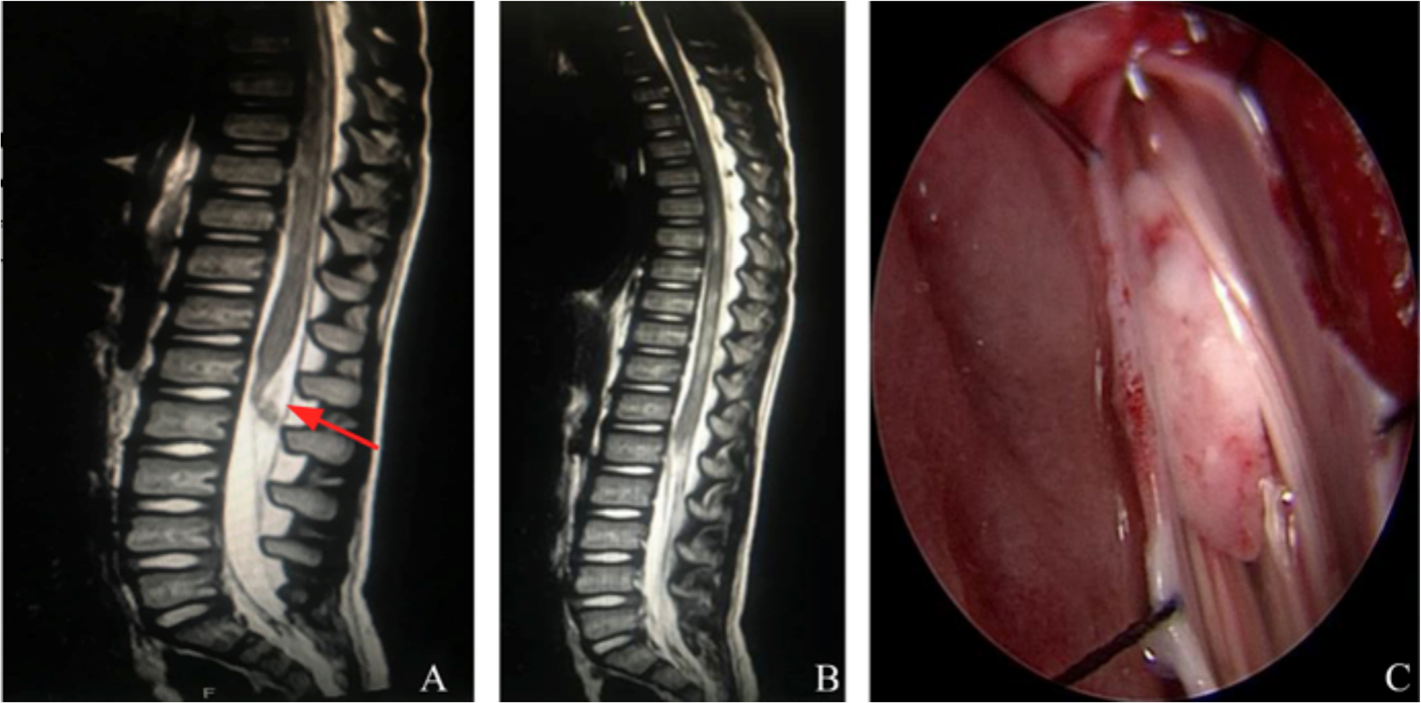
a A nodular T2 isointensity shadow was showed at the dorsal of the conus, which was located at L1 level with moderate swelling. b The sagittal MRI images showed longitudinally extended intramedullary patchy T2 hyperintensity at the T9-L1 level. c There were contusion and laceration in the cone and some contusion and laceration tissue outflew the soft spinal meninges
